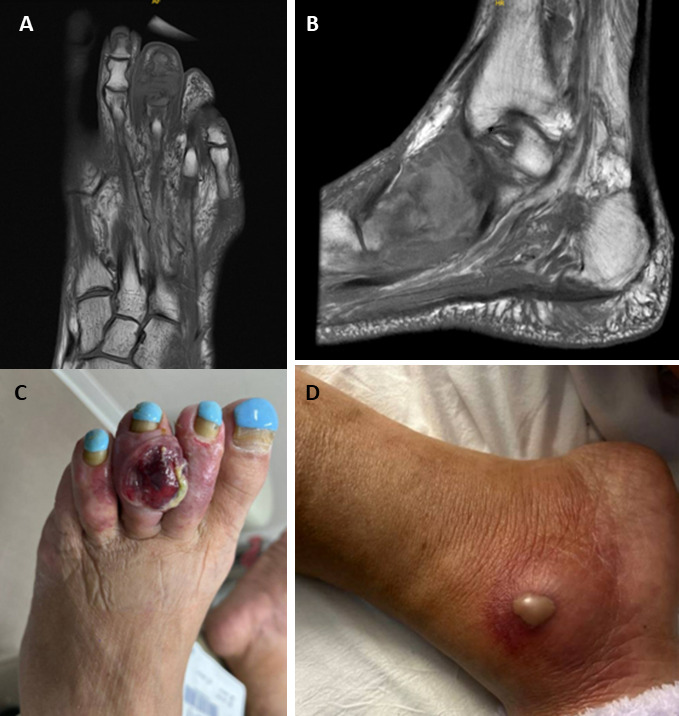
(A) MRI of left foot showing third toe soft tissue mass and osseous destruction of middle phalanx and distal phalanx base. (B) Left third toe lesion. (C) MRI of right foot showing large heterogeneous mass with extensive destruction of adjacent bones. (D) Right ankle lesion.
